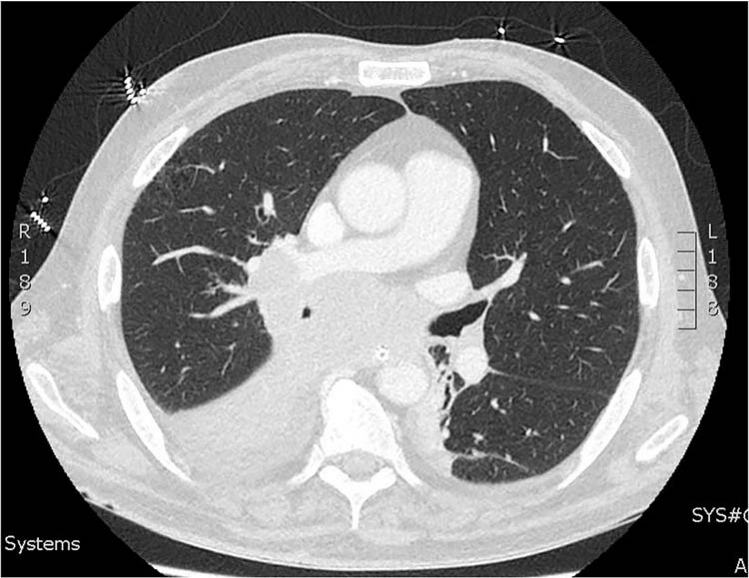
CT of the chest from day two admission demonstrating soft tissue mass enveloping the right bronchi.
